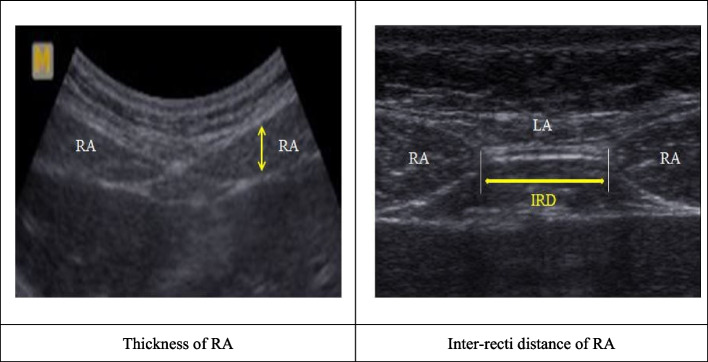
Measurement of inter-recti distance and thickness of rectus abdominis by ultrasound. RA: Rectus abdominis, LA: Linea alba, IRD: Inter-recti distance
